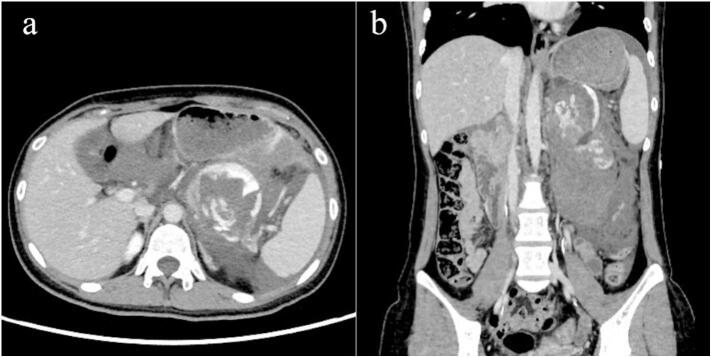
(a) Contrast-enhanced computed tomography (CT) showing contrast blush in the cyst, which delineates the margin of the cyst. (b) Coronal CT showing a retroperitoneal hematoma spreading caudally with concurrent flow into the retroperitoneum, in addition to an intra-cystic hemorrhage.
